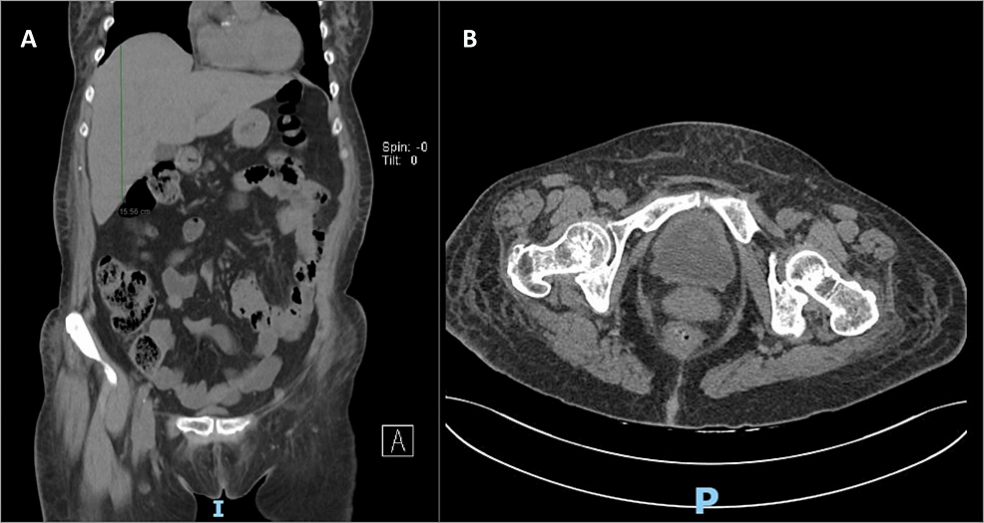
Abdominal CT scan. A: Hepatomegaly. B: Bladder diffuse thickness.
